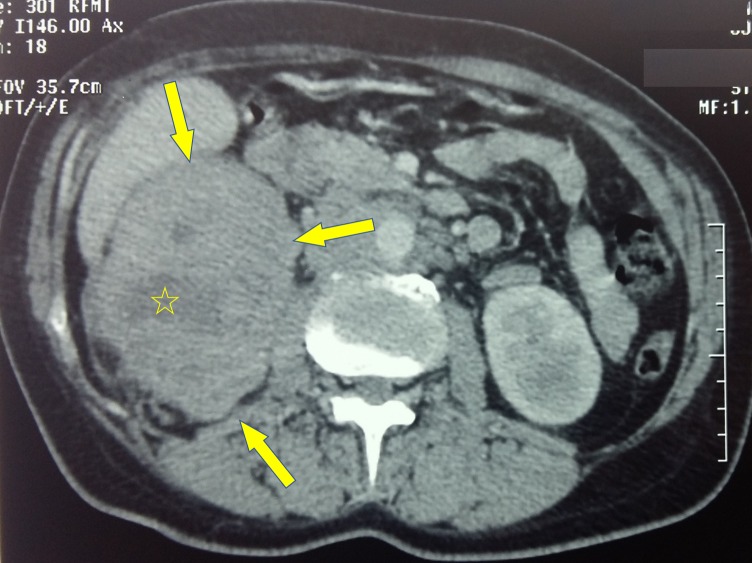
Right renal mass Axial CT scan showing large right renal mass (denoted by arrows), poorly defined, heterogeneous, and containing hypodense regions of tumor necrosis (denoted by the star). CT, computed tomography.
